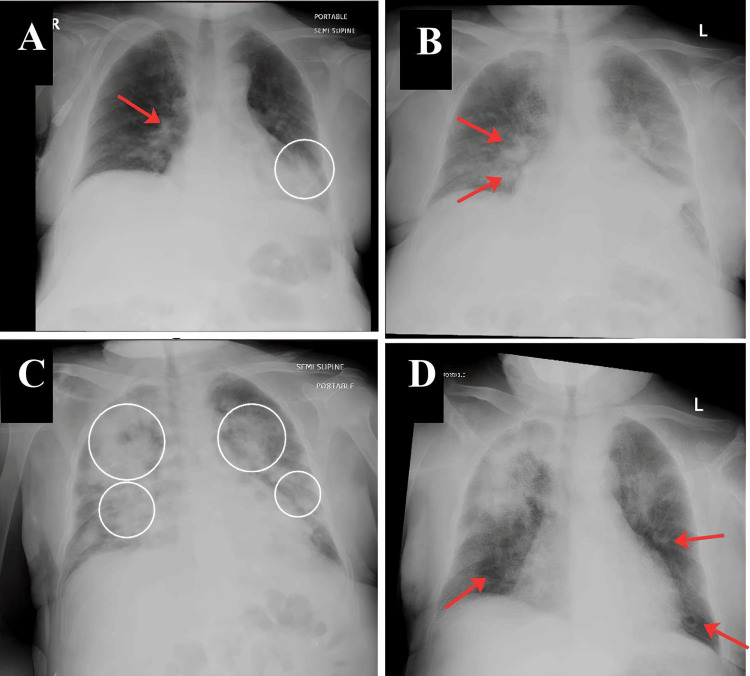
Serial portable chest radiographs show the progression and resolution of daptomycin-induced eosinophilic pneumonia (A) A baseline chest radiograph on admission (hospital day 0) shows prominent bronchovascular markings (red arrow) and left lower-zone atelectatic changes without focal consolidation (circle). (B) A chest radiograph on hospital day 37 (day 24 of daptomycin therapy) shows newly developed opacity in the right middle and lower lung zones (red arrows). (C) A chest radiograph on hospital day 40 shows progression to bilateral patchy air-space opacities (circles). (D) A chest radiograph on hospital day 43, 3 days after daptomycin discontinuation, shows improved aeration and near-complete resolution of infiltrates (red arrows).
